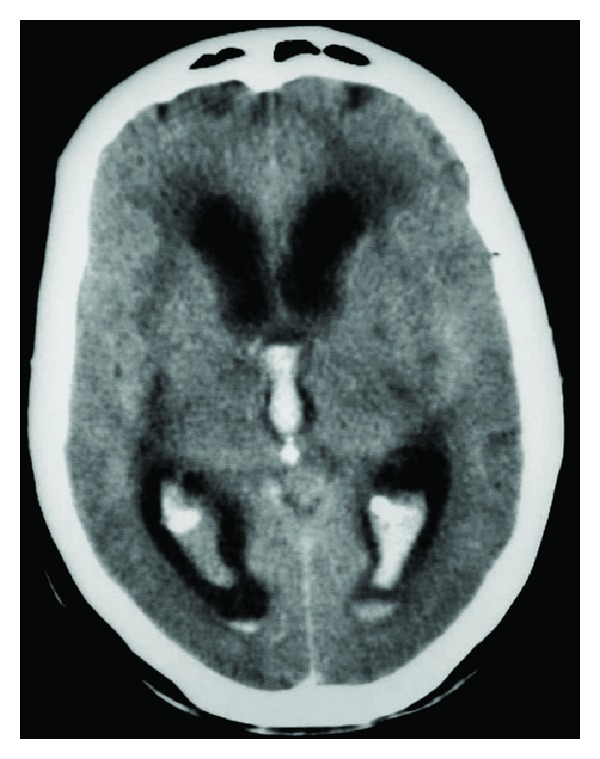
Primary intraventricular hemorrhage in the brain CT scan.
